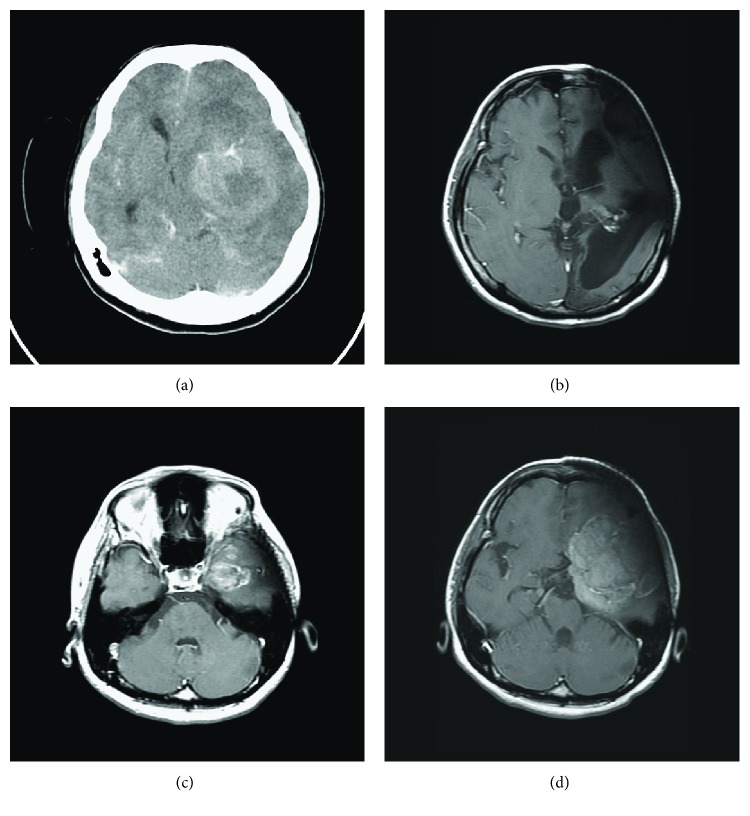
(a) Brain computed tomography in December 2006 showed an enhanced brain tumor over the left temporal region with mass effect; (b) no obvious recurrent tumor was found by brain MRI in 2013; (c) recurrent tumor was found by MRI in 2014, before the second surgery; and (d) brain MRI in May 2015, before the third surgery, revealed tumor progression.
